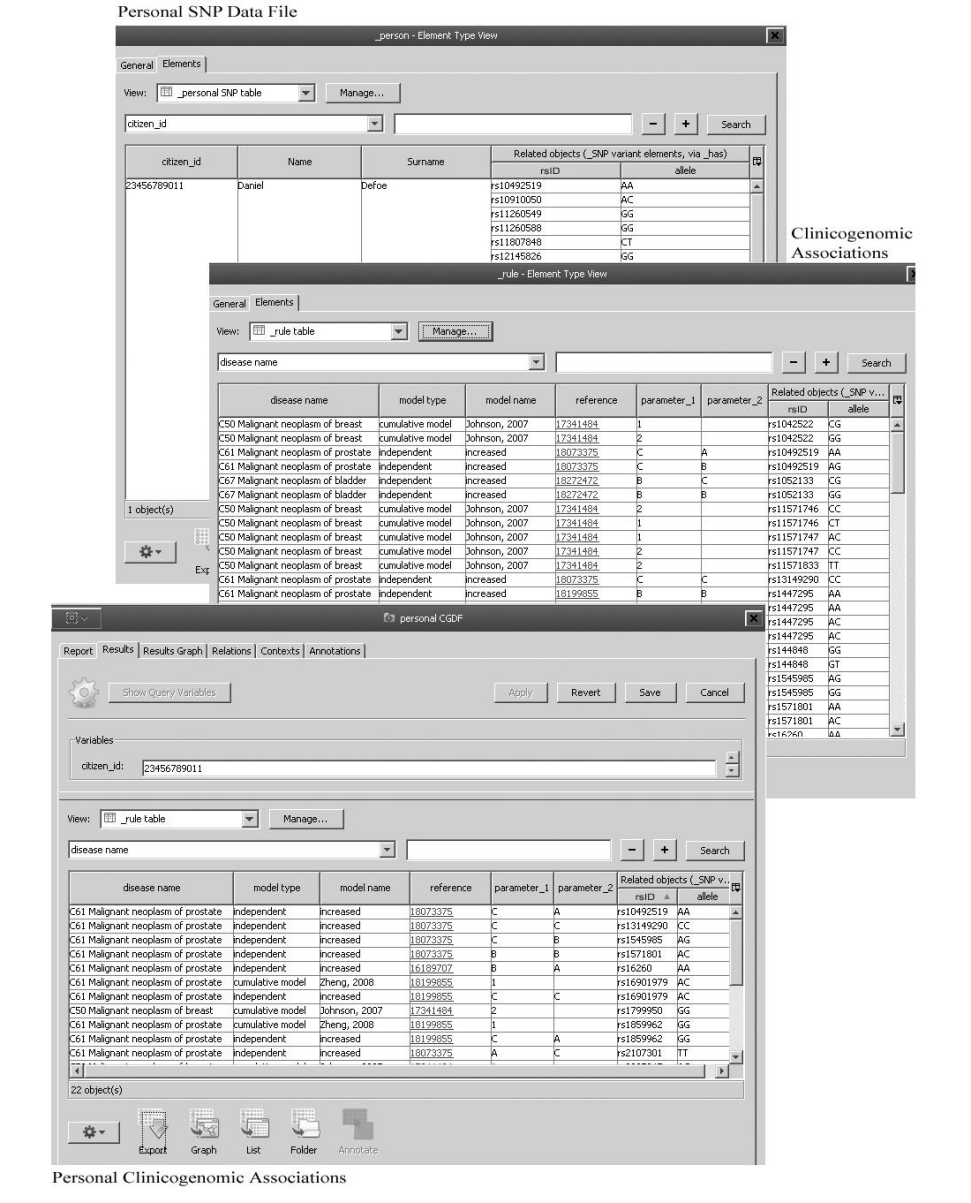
Functional steps for Clinicogenomic Knowledge Base (ClinGenKB): (1) defining clinicogenomic content, (2) uploading personal clinically relevant single nucleotide polymorphism (SNP) data, and (3) inferring personal clinicogenomic associations based on the content. rsID=reference single nucleotide polymorphism identifier; AA=adenine-adenine; AC=adenine-cytosine; AG=adenine-guanine; CC=cytosine-cytosine; CG=cytosine-guanine; CT=cytosine-thymine; and GG=guanine-guanine.
